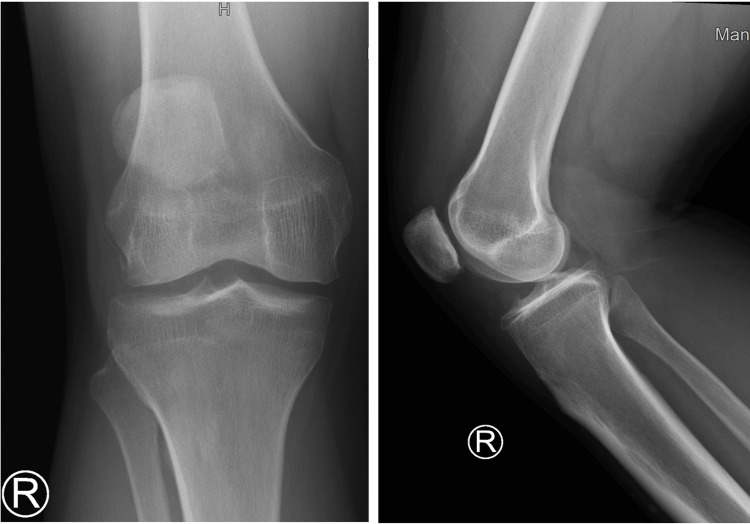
X-ray AP and lateral views of the knee joint show moderate osteoarthritis.
